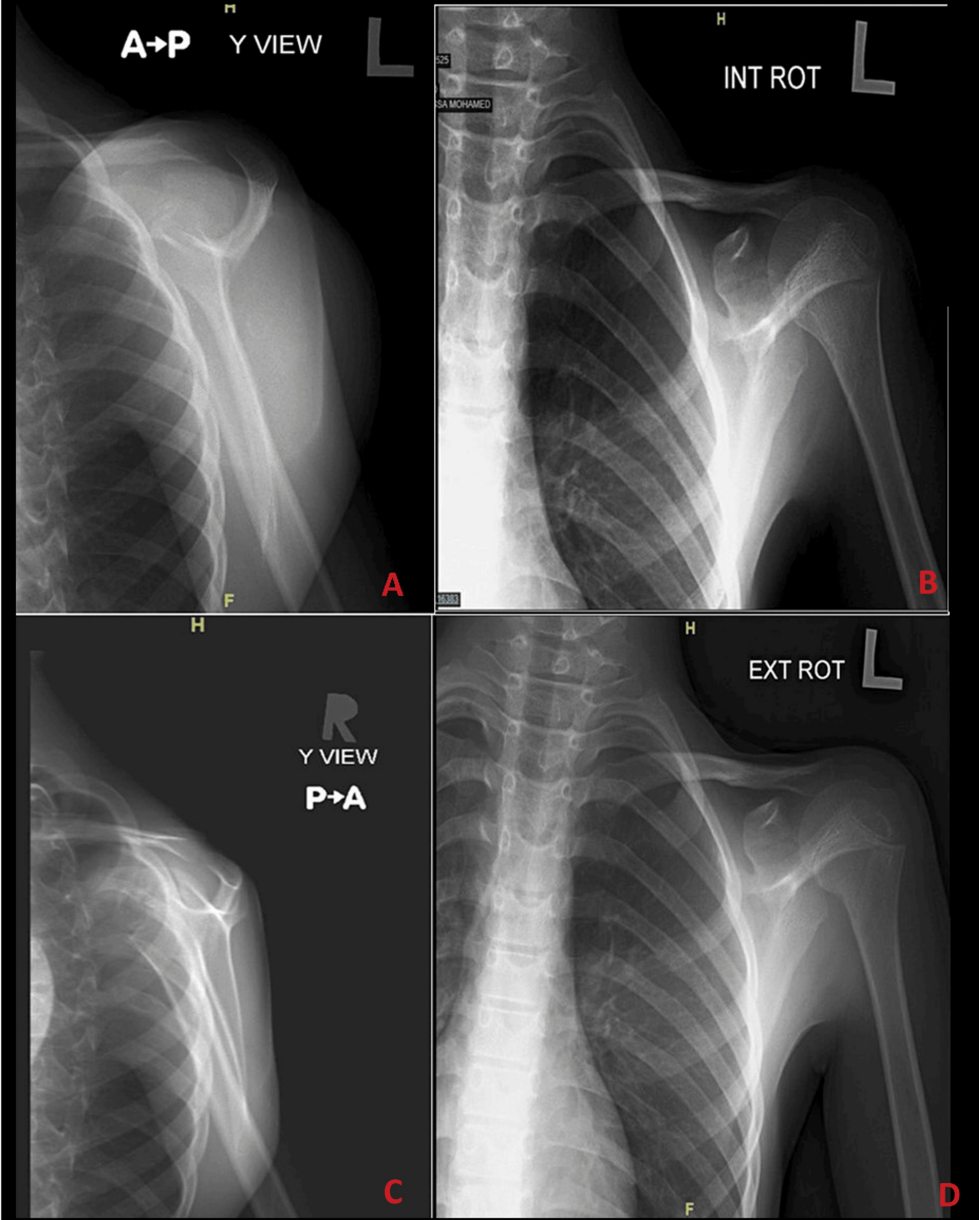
X-rays of the left shoulder showing significant soft-tissue thickening and swelling around the left scapula, with preserved alignment and joint spaces: (A) anteroposterior view of left shoulder; (B) internal rotation view of left shoulder; (C) Y-view of left shoulder; and (D) external rotation view of left shoulder
